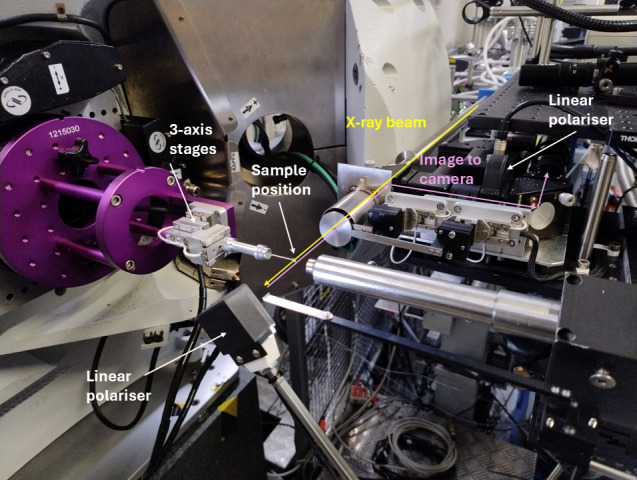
Photograph of the experimental setup at I19 at the UK Synchrotron Diamond Light Source, showing the sample in the X-ray path, the camera setup to take in-line photographs of the sample and the position of the polarizers for capturing birefringence.
